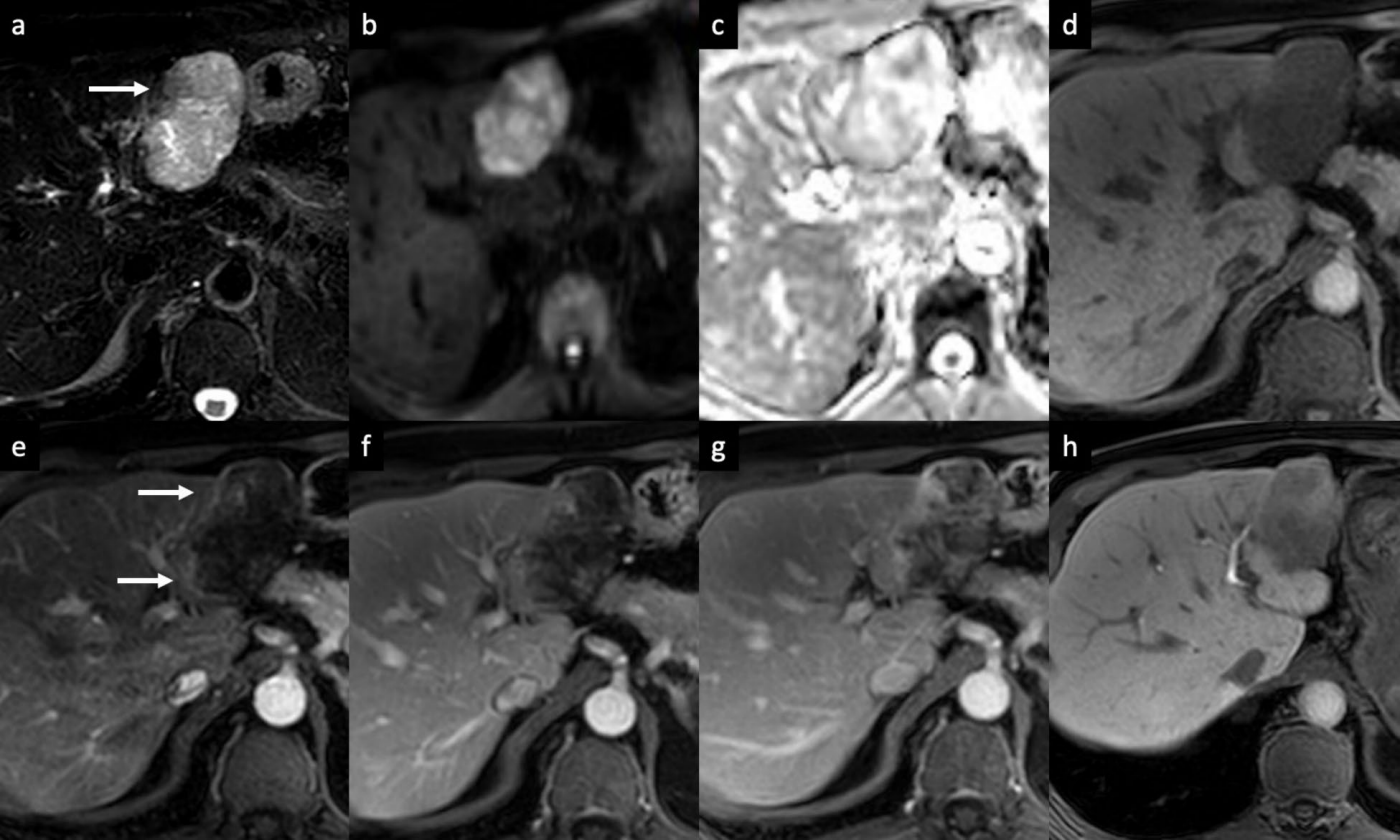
Sclerosed hemangioma in a 69-year-old man. MRI shows a focal liver lesion in the left lobe with heterogenous high signal intensity on axial fat-suppressed T2-weighted image (arrow, a), with high signal intensity on diffusion-weighted imaging (b), and high intensity on the apparent diffusion coefficient map (c). On contrast-enhanced MR images the lesion shows low signal intensity on unenhanced T1-wighted image (d), and peripheral discontinuous enhancement on arterial phase (arrows, e), followed by a progressive centripetal enhancement on portal venous (f) and delayed (g) phases. On the hepatobiliary phase acquired 2 h after the administration of gadobenate dimeglumine contrast agent, an heterogenous low signal intensity is observed
